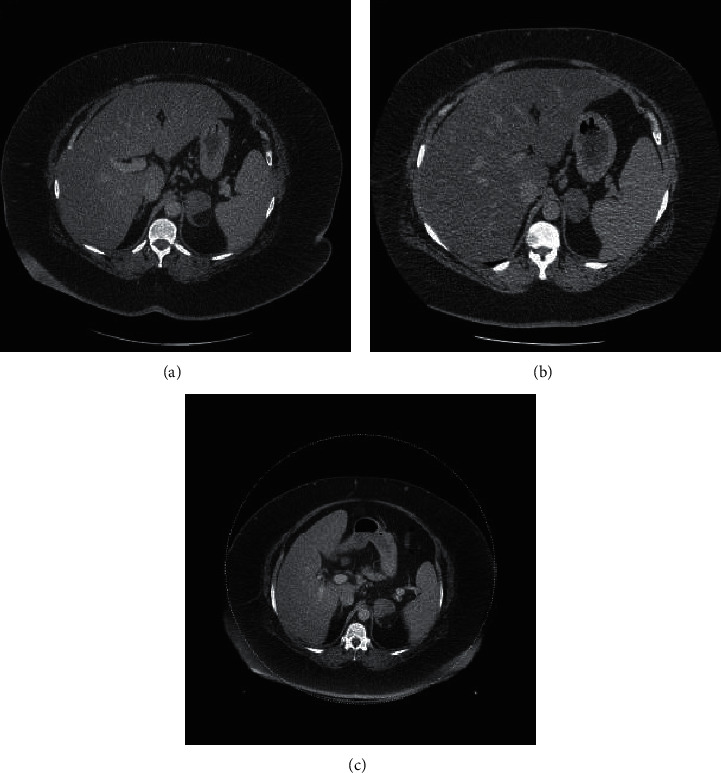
A CT for abdominal pain in November 2012 revealed a presumed adrenal myelolipoma with a focus of macroscopic fat (a). In comparing to a prior exam from April 2007 (b), this mass had grown in size, and subsequent imaging from April 2016 (c) continued to demonstrate growth.
